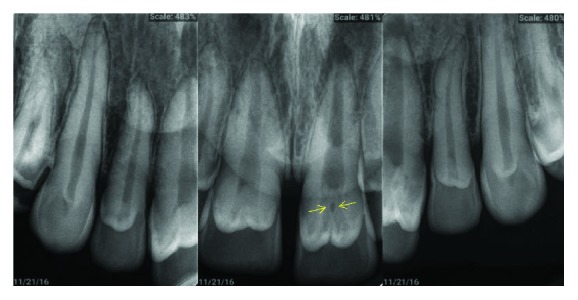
Case 1: periapical radiograph shows talon cusps in all upper anterior teeth. Notice the small radiolucent teardrop in the centre of the crown of the left central incisor (dens invaginatus).
